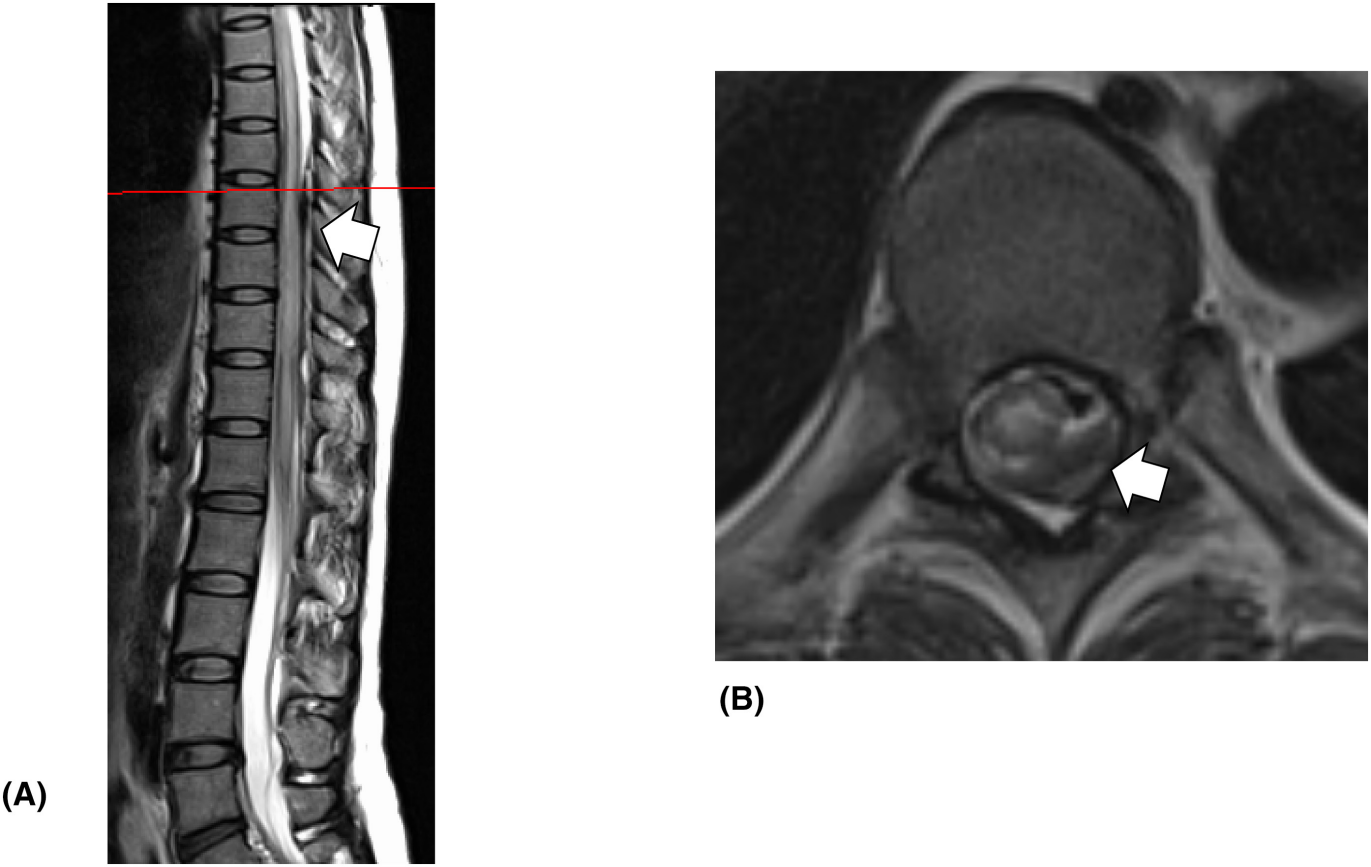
Magnetic resonance imaging (MRI) before surgery. (A) Sagittal T2‐weighted MRI showing spinal cord epidural hematoma at the T8–L1 level, with T9 displaying the area with the most severe spinal cord compression (white arrow) and extensive spinal cord edema. (B) Axial T2‐weighted MRI showing significant left‐sided spinal cord epidural hematoma at T9 level.
